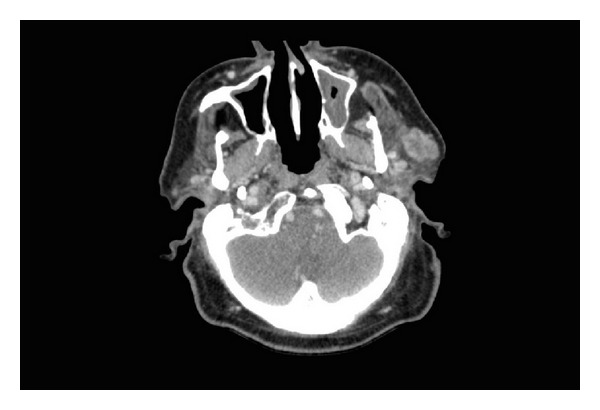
Preoperative CT showing parotid gland lesion.
